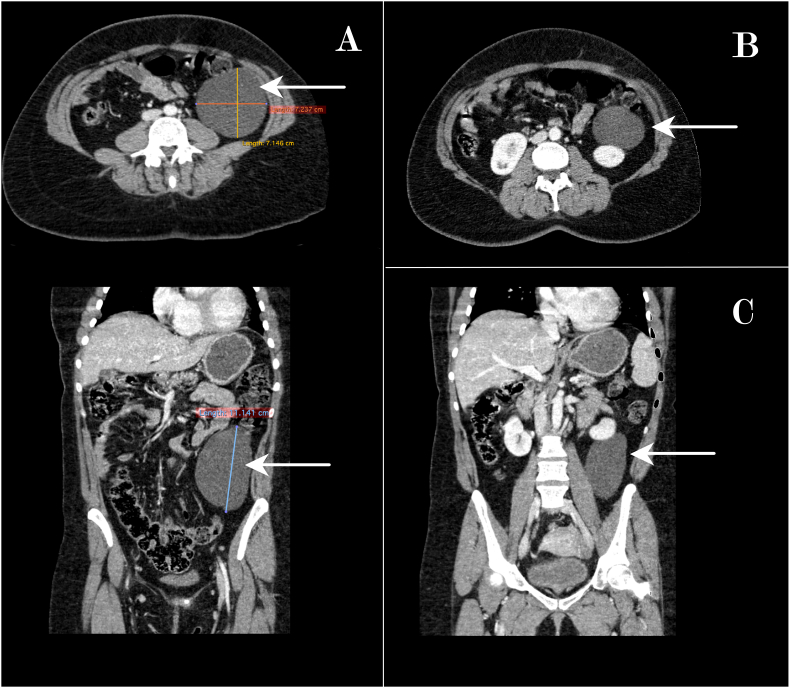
Large retroperitoneal cystic mass: Axial and coronal view of Abdominal computed tomography (CT) scan showing a cyst *(arrow)* with a thin wall and a homogenous liquid content and measuring 72x71x112 mm (A). The cyst exerts mass effect on the descending colon (B) and the left kidney (C).
